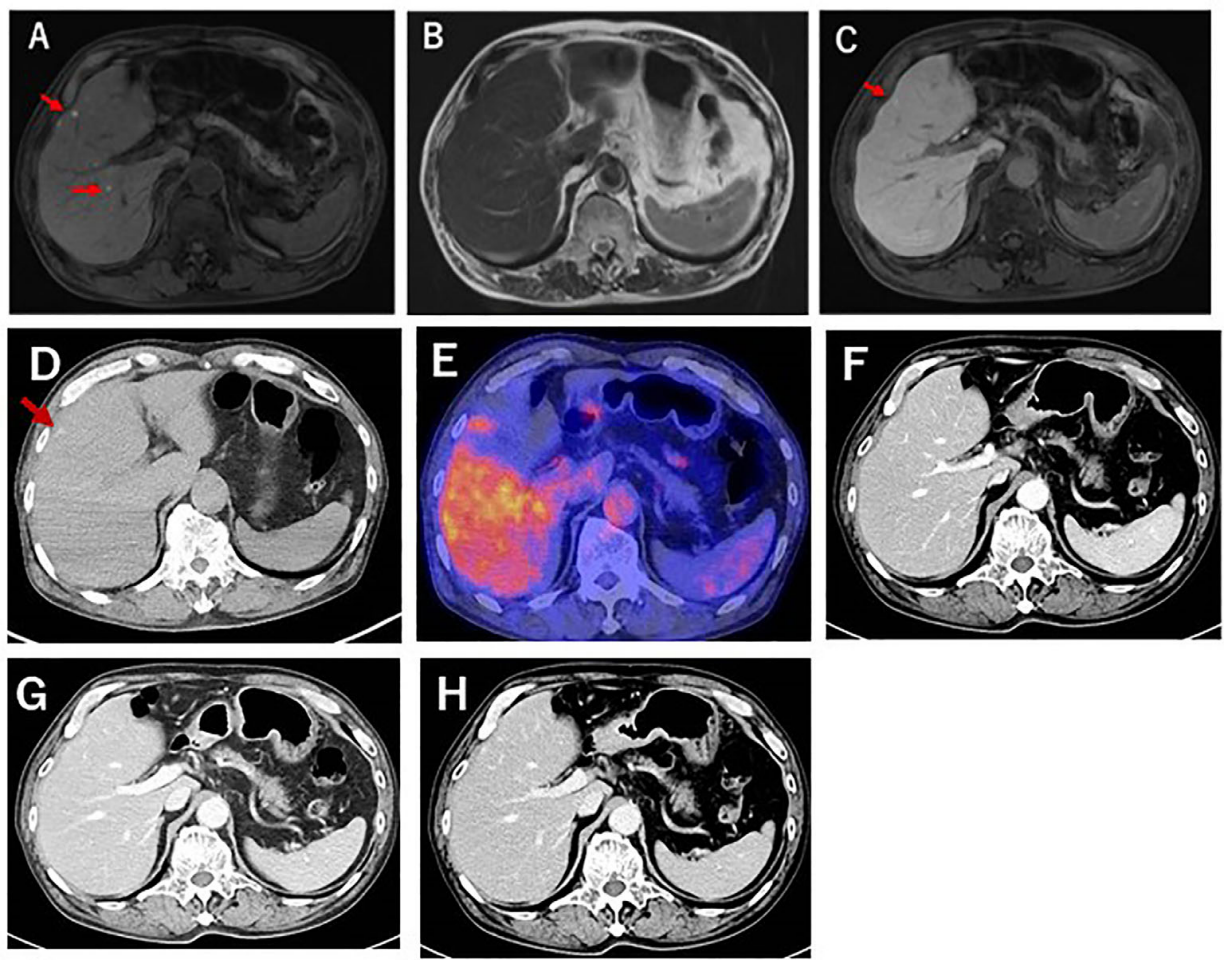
Gd-EOB-DTPA enhanced MRI of this patient. (A) T1-weighted MRI showing that the liver nodules (red arrows) were highly intense. (B) T2-weighted MRI, showing that the liver nodules were iso-intense. (C) Gd-EOB-DTPA-enhanced MRI, showing the showed were highly intense during the hepatobiliary phase. (D) Plain-CT examination 1 year before liver biopsy, showing small, high-density nodules. (E) FDG-PET CT examination at liver biopsy. No abnormal FDG uptake was detected. (F–H) Dynamic CT examination during the (F) arterial, (G) portal phase, and (H) equivalent phases. No enhancement was detected.
